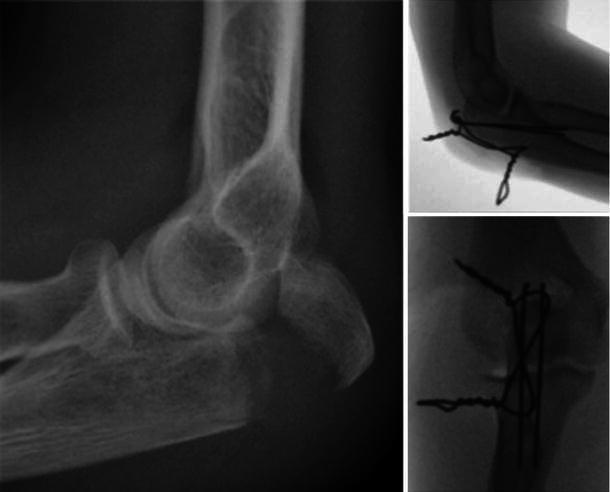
Radiograph showing simple transverse olecranon fracture (*left*), intra-operative image intensifier view showing good fracture reduction
